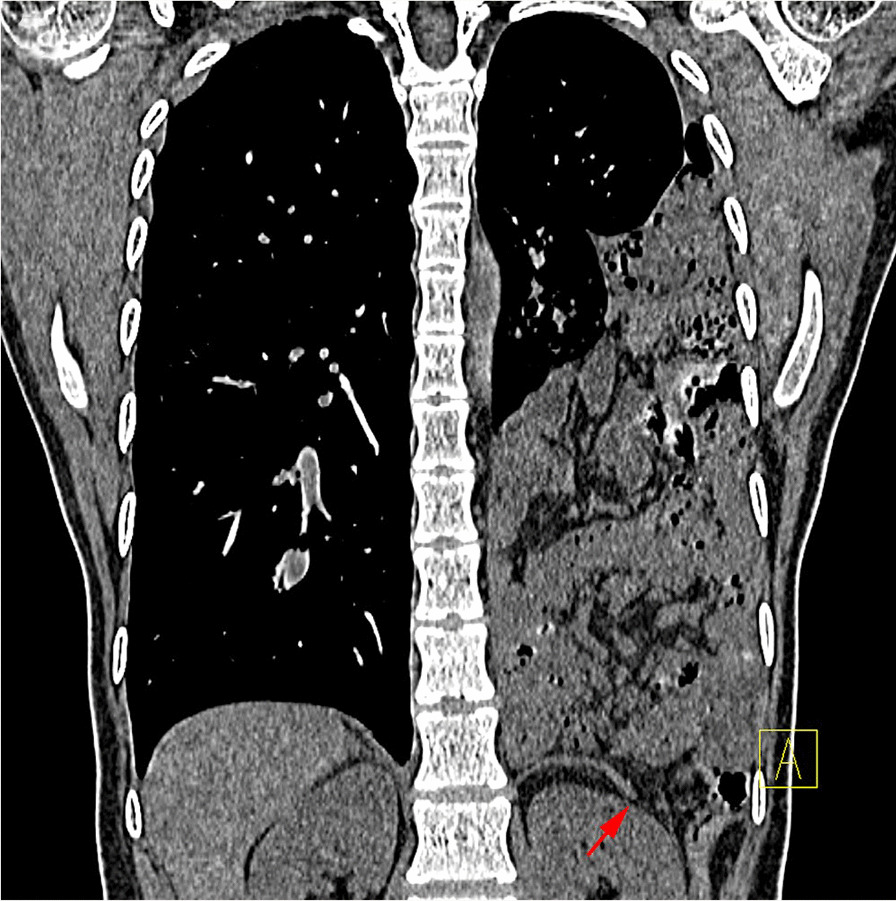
Computed tomography images showing the bowel loops and fat passing through a defect in the posterolateral left hemidiaphragm (arrow)
